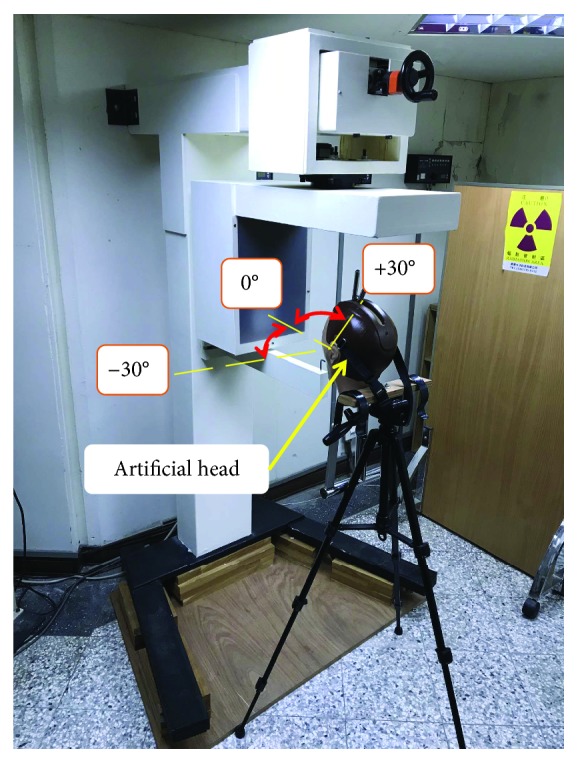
2.5D periapical radiography system and X-ray tube scanning ranges.
